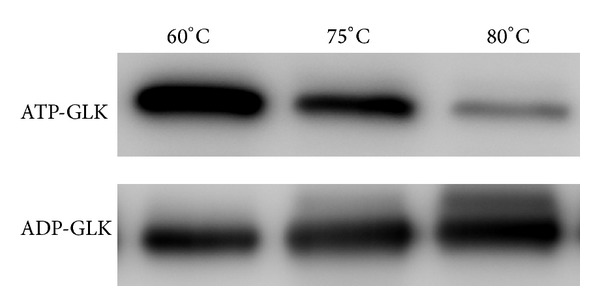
Western blot analysis of ATP- and ADP-GLK at different temperatures. *T*. *tengcongensis* cells cultured at different temperatures were collected and disrupted by ultrasonication. Equal amounts of *T*. *tengcongensis* soluble protein lysate (20 g) were loaded for SDS-PAGE separation followed by Western blot using antibodies against ATP- and ADP-GLK, respectively.
